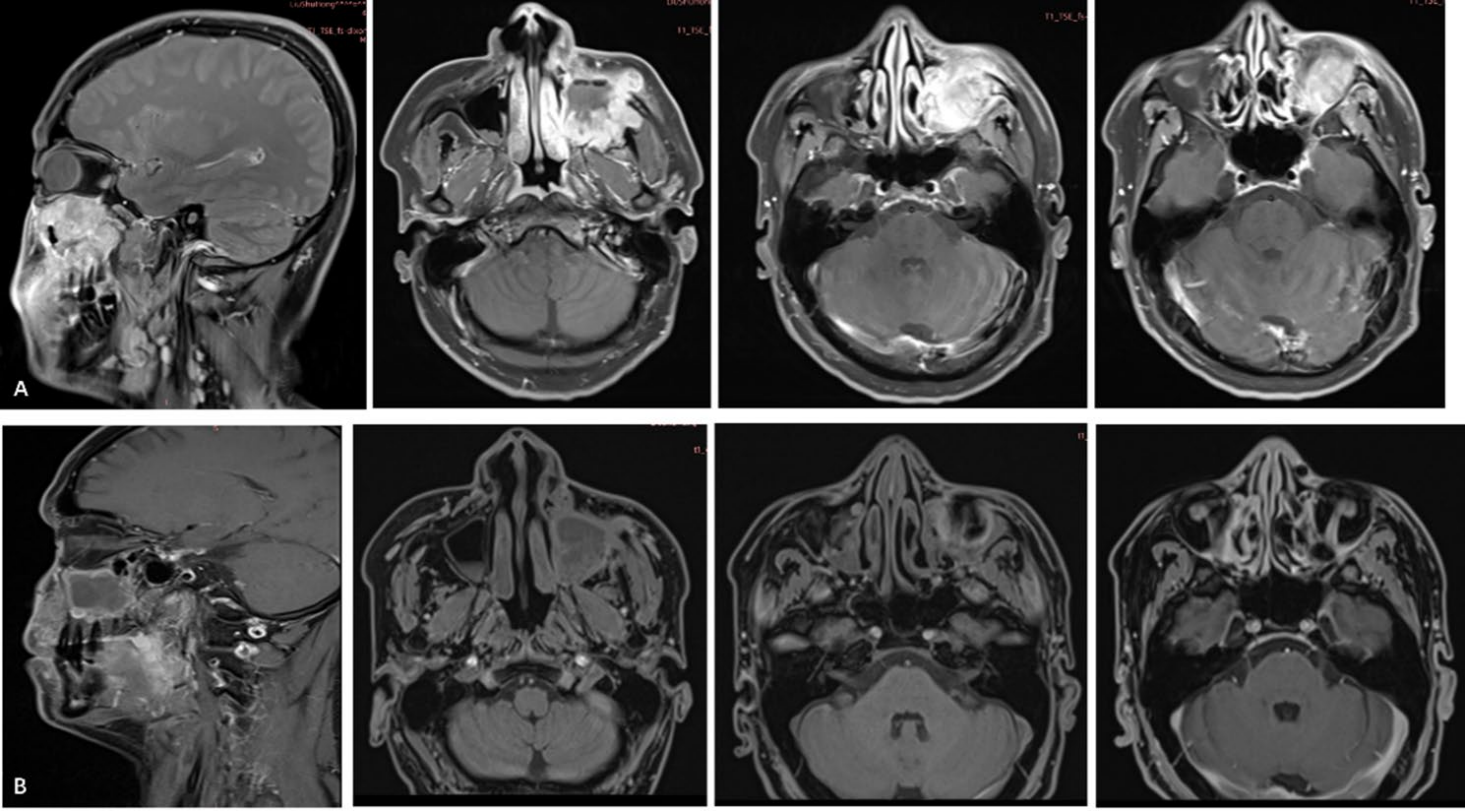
Radiological response assessment. A MRI (March 8, 2021) showing tumor compression of the optic nerve. B MRI (May 19, 2021) revealing a clear fat plane between the residual lesion and the optic nerve
